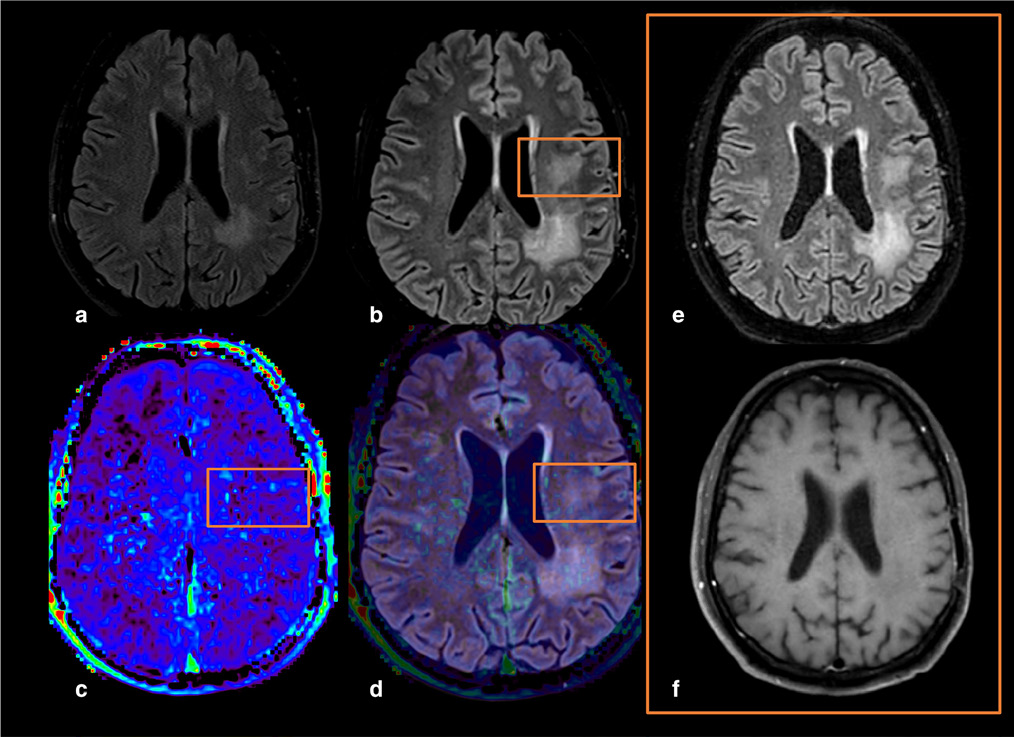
Example of a glioblastoma (IDH-wildtype, WHO grade 4) five months (a) and seven months after STUPP protocol (b), showing the appearance of a non-contrast FLAIR hyperintensity in the frontal left corona radiata (little orange rectangle) that didn’t show any increase in APTw signal intensity, therefore suggesting a non-tumoral, post-therapeutic (probable radiation-induced leukoencephalopathy) lesion, as confirmed by follow-up examination (e-f). (a,b) 3D FLAIR images at different time points (five and seven months after STUPP). (c) F.S. APTw image. (d) F.S. APTw map, superposed to FLAIR image, (e,f) two-months follow-up after B, with 3D FLAIR (e) and 3D T1w after injection (f) that confirm the lesion stability with no contrast enhancement, and therefore strongly suggest then non-tumoral nature of the lesion. 3D, three-dimensional; ADC, apparent diffusion coefficient; APTw, amide proton transfer-weighted; FLAIR, fluid attenuated inversion recovery.
